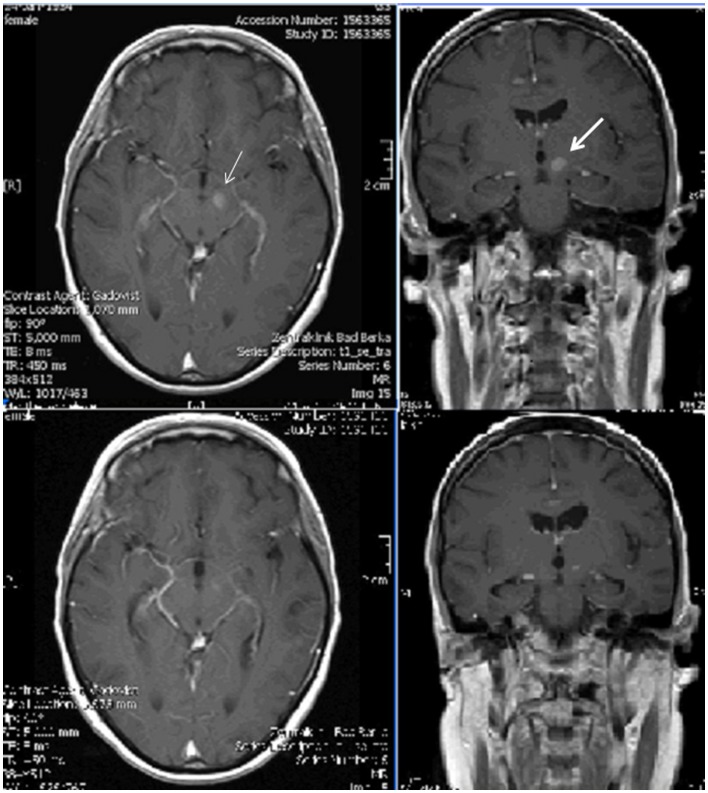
Top: T1-weighted gadolinium-enhanced axial and coronal magnetic resonance images before brain radiation therapy: lesions on the left side of the mesencephalon (left, thin arrow) in the left thalamus (right, thick arrow); bottom: corresponding images after therapy presenting much less clear lesions.
